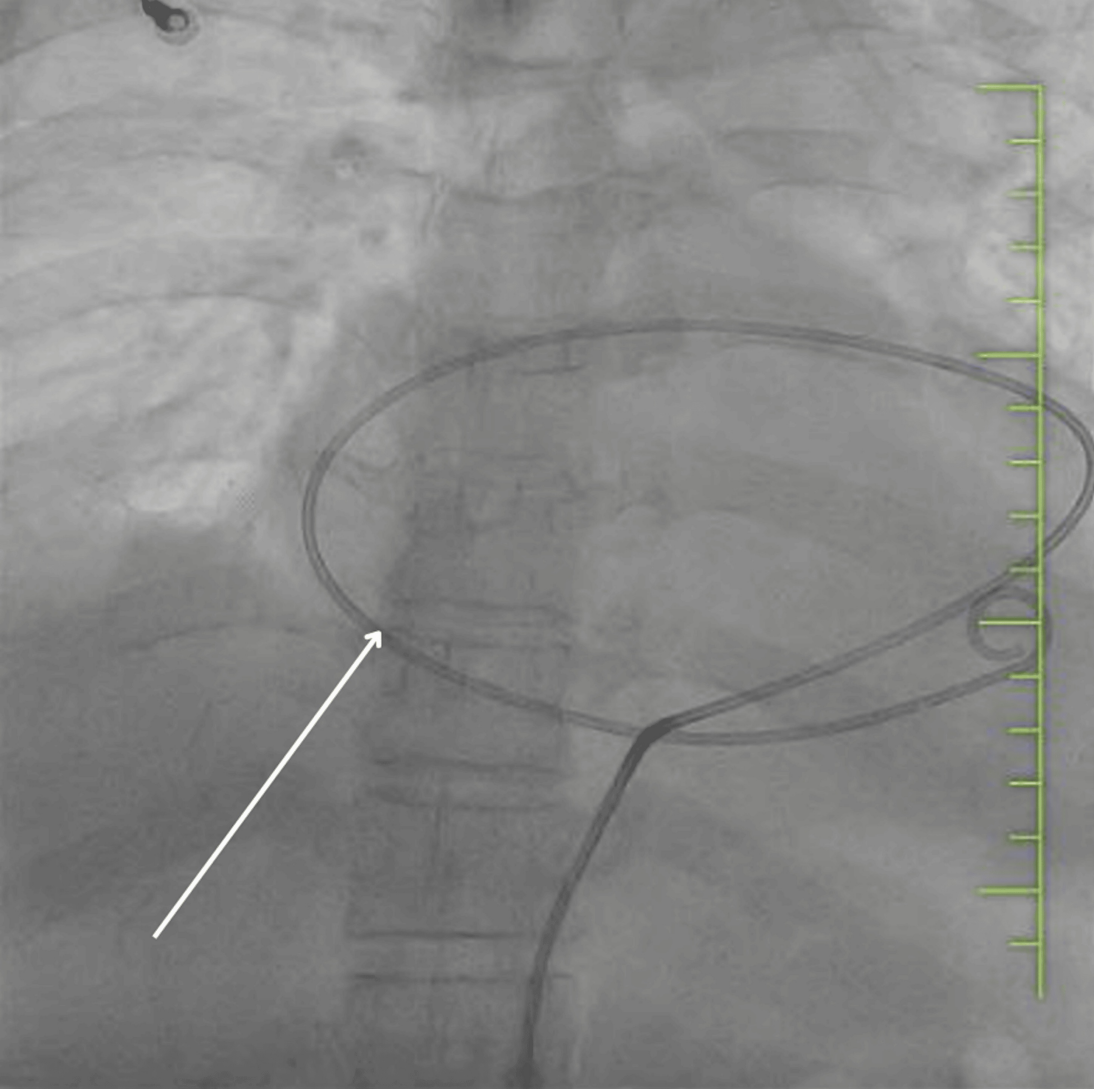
Chest radiograph with pericardial drain indicated by white arrow
